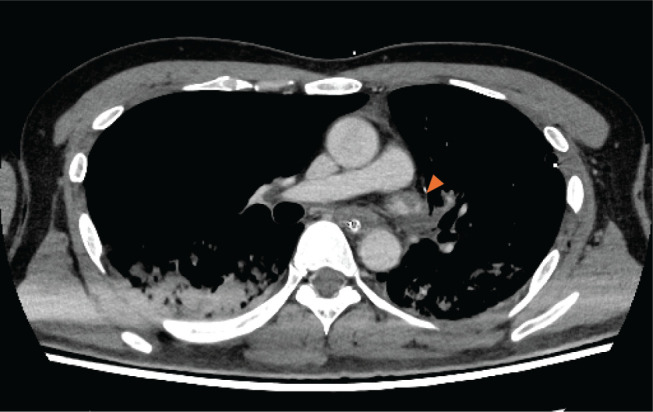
Thromboses on CT. Contrast CT immediately after the return of spontaneous circulation suggested thrombosis of the pulmonary vein stump. Arrowhead, pulmonary vein stump
